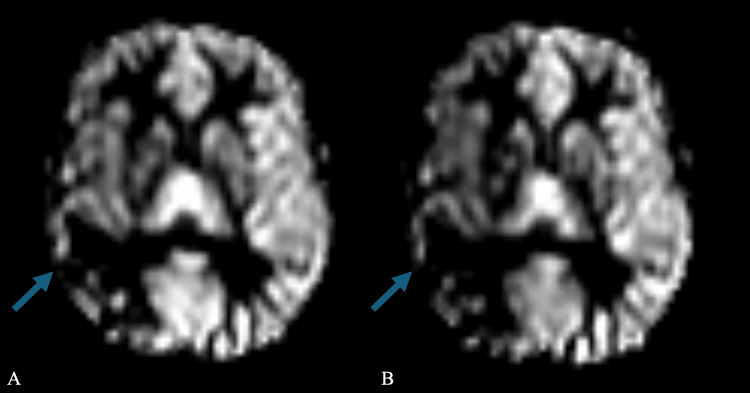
MRI pre-Diamox (A) showed decreased blood flow in the right middle cerebral artery territory, and post-Diamox (B) demonstrated further decreased flow in the same right middle cerebral artery territory, suggestive of maximal flow compensation with poor vascular reserve
